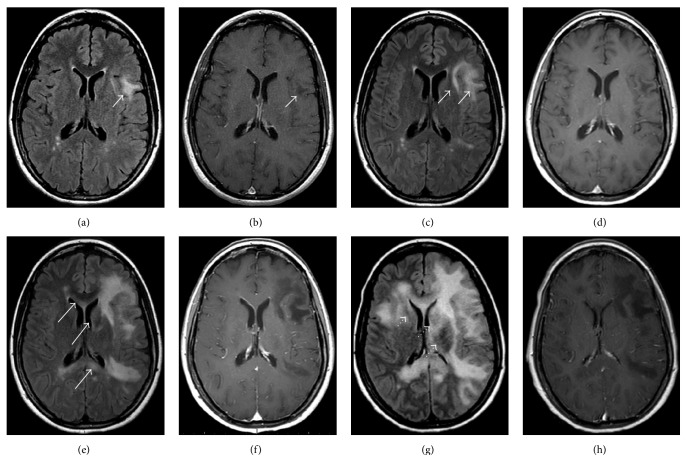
32-year-old woman with RRMS and no new neurologic symptoms developed MR findings on FLAIR images (a) without enhancement (b) consistent with PML (arrows) after having been on natilizumab for approximately 4.5 years. The drug was discontinued and she received PLEX and steroids. Follow-up imaging was obtained at (c-d) one month, (e-f) three months, and (g-h) four months later demonstrating progressive PML lesions, which corresponded to progressive clinical neurological decline. FLAIR (c) and postcontrast (d) images obtained at one month demonstrate disease progression without enhancement. Images obtained at three months show progressive disease (e-f) without enhancement to suggest active MS or IRIS. Like other aggressive infiltrating white matter lesions PML can cross the corpus callosum (arrows). Note also that there is now involvement of the left caudate (dotted arrow). The final images obtained four months after presentation (g-h) demonstrate swelling and compression of the gyri. There is now marked involvement of the deep gray matter structures (dotted arrows) which occurs in up to one-third of cases. However, note that despite involvement of almost the entire hemisphere, the lateral ventricle remains only mildly compressed and there is no midline shift, as would be expected with other lesions of this size, and no enhancement has developed.
